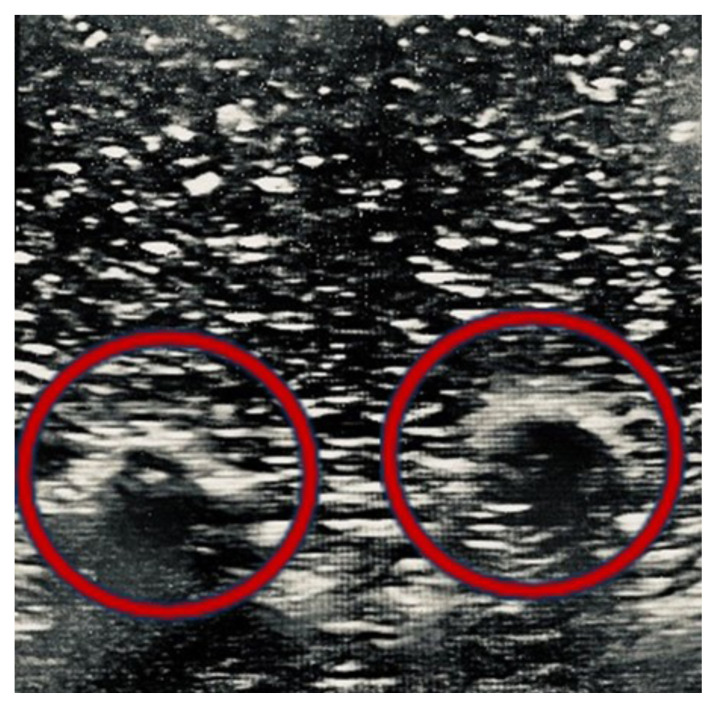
Author’s own image. Ultrasound image of ribs on the created model (red circles).Next, the needle was advanced in-plane until successfully arriving at the targeted rib.[Fig f9-jetem-10-2-i1]Figure 9 f9-jetem-10-2-i1:
